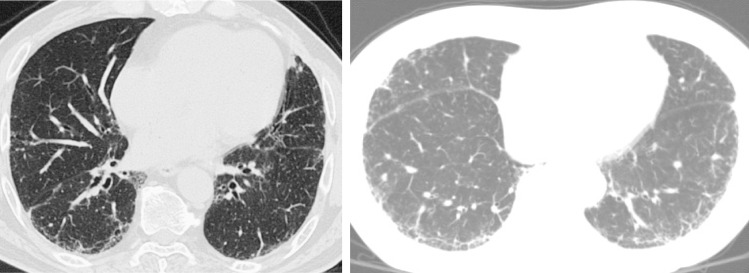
HRCT images of two patients with representative HRCT scans, showing reticular abnormalities and/or subpleural irregularities without honeycombing.
